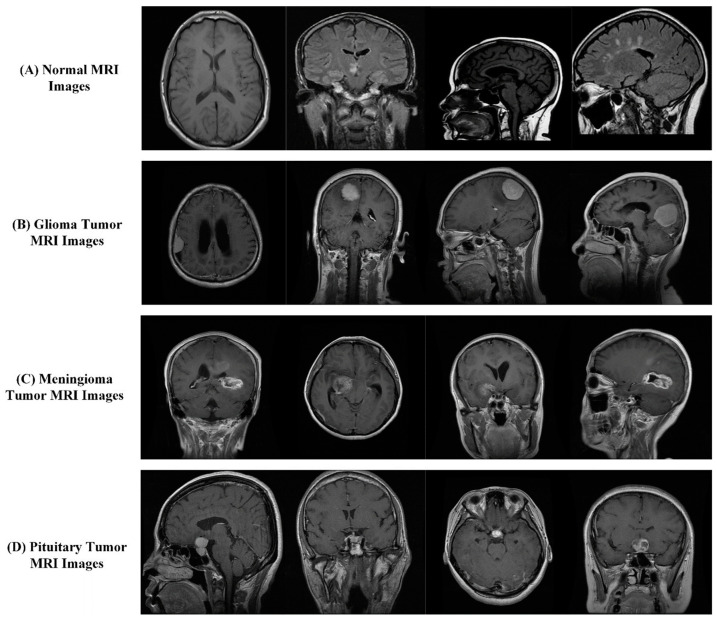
Example MRI images of normal and different types of tumors.
